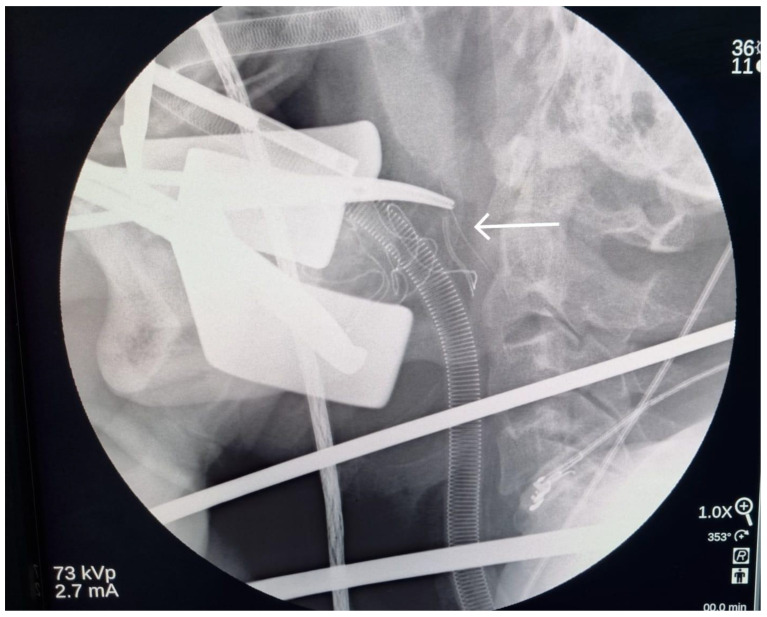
Multiple X-ray images (lateral neck view) were taken to insure the adequate depth of dissection. When the adequate depth was achieved, the angled hemostat was placed just posterior to the needle on lateral neck view, and a postero-anterior neck X-ray was taken to identify the fractured needle location on the vertical plan. It was just lateral to the hemostat (arrow), which was placed in medial pterygoid-superior constrictor plane. The hemostat was slightly withdrawn and directed to dissect carefully through the medial pterygoid muscle until a part of the fractured needle was located with the assistance of the endoscope.
